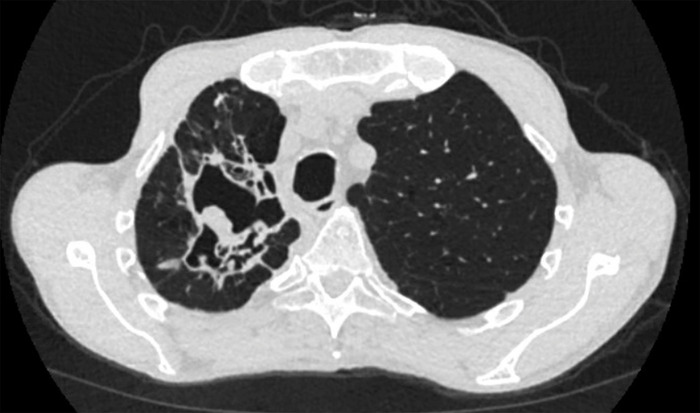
Follow-up chest computed tomography after 5 weeks, transverse view of lower lobes, shows near complete clearing of bibasilar opacities. Bilateral partially calcified nodules and amorphous/nodular opacities (more on the right than on the left) are visible, as well as bilateral bronchiectasis (also more on the right than on the left).
